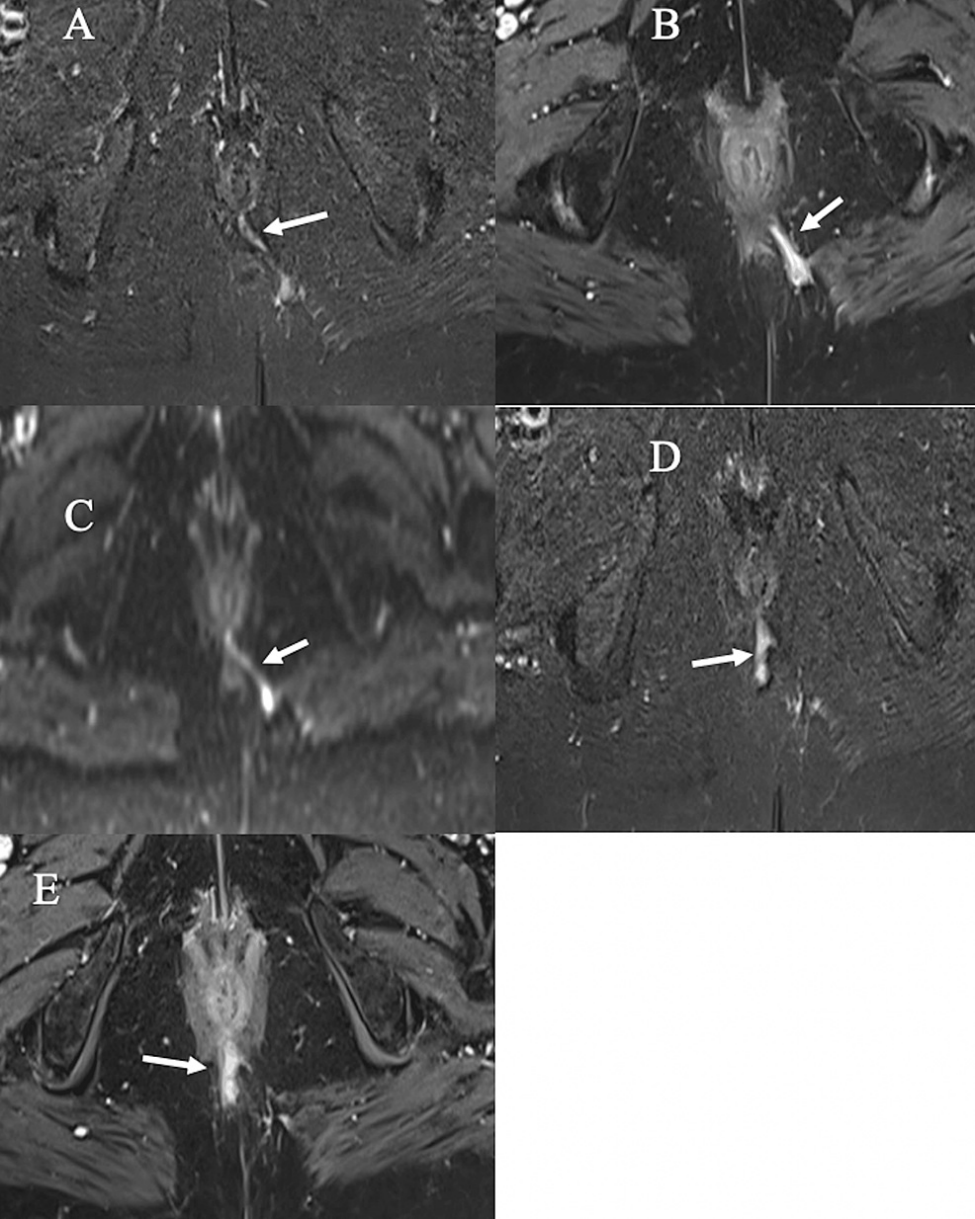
Grade 4 trans-sphincteric fistula with secondary ramification. (A-C) Axial STIR, post-contrast fat-suppressed T1-WI and DWI, respectively, showing grade 4 trans-sphincteric fistula crossing the external sphincter with a suspicious internal opening at the 6 o’clock position with restricted diffusion on DWI. (D-E) Axial STIR and post-contrast fat-suppressed T1, respectively, show secondary ramification coursing posteriorly. DWI: diffusion-weighted imaging, STIR: short tau inversion recovery
